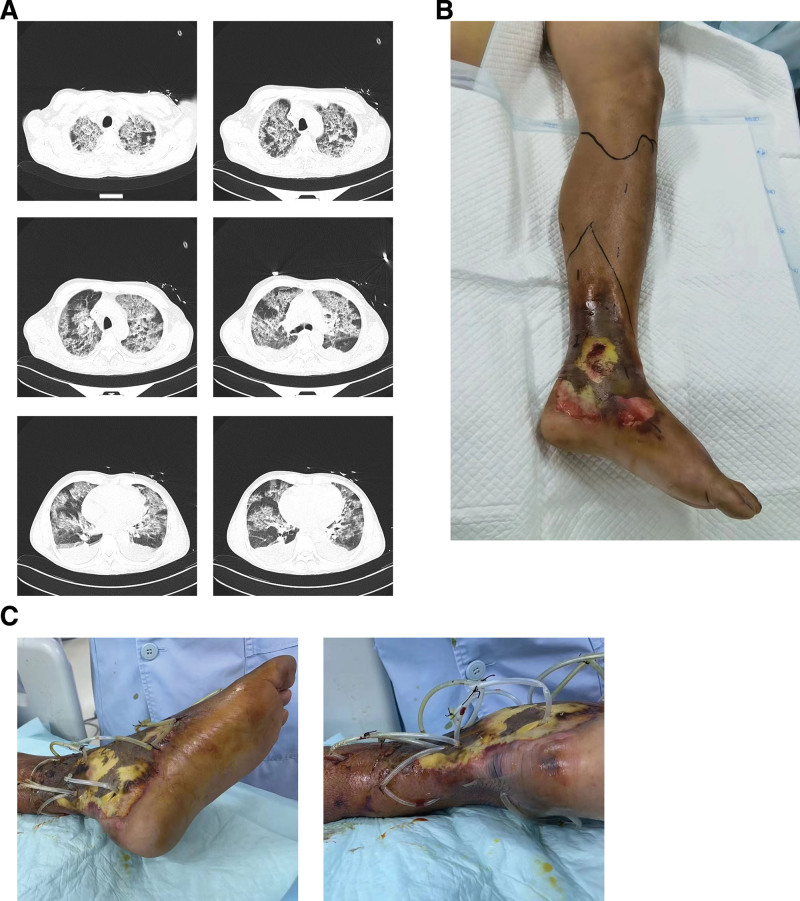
(A) Chest computed tomography (CT) on the 7th day of the patient. (B) Skin infection and tissue necrosis of the left lower. (C) Incision and drainage surgery for infection of the left.
